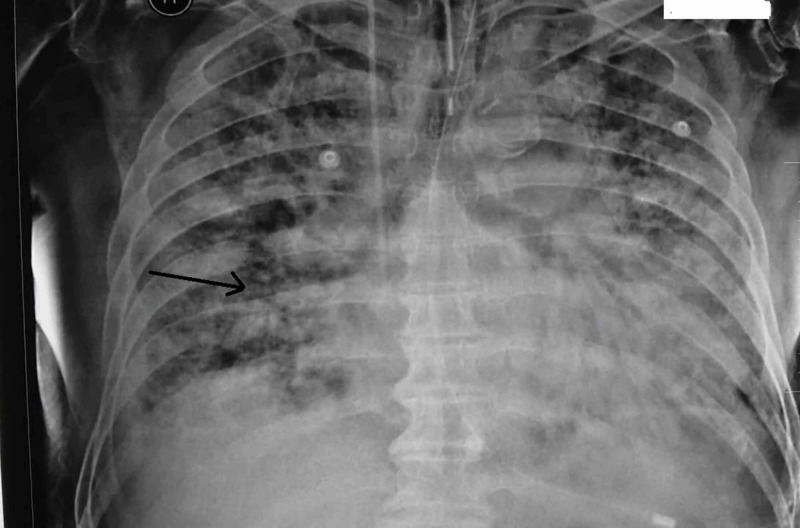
Chest X-ray PA view revealing a severe ARDS. PA: Posteroanterior; ARDS: Acute Respiratory Distress Syndrome.
